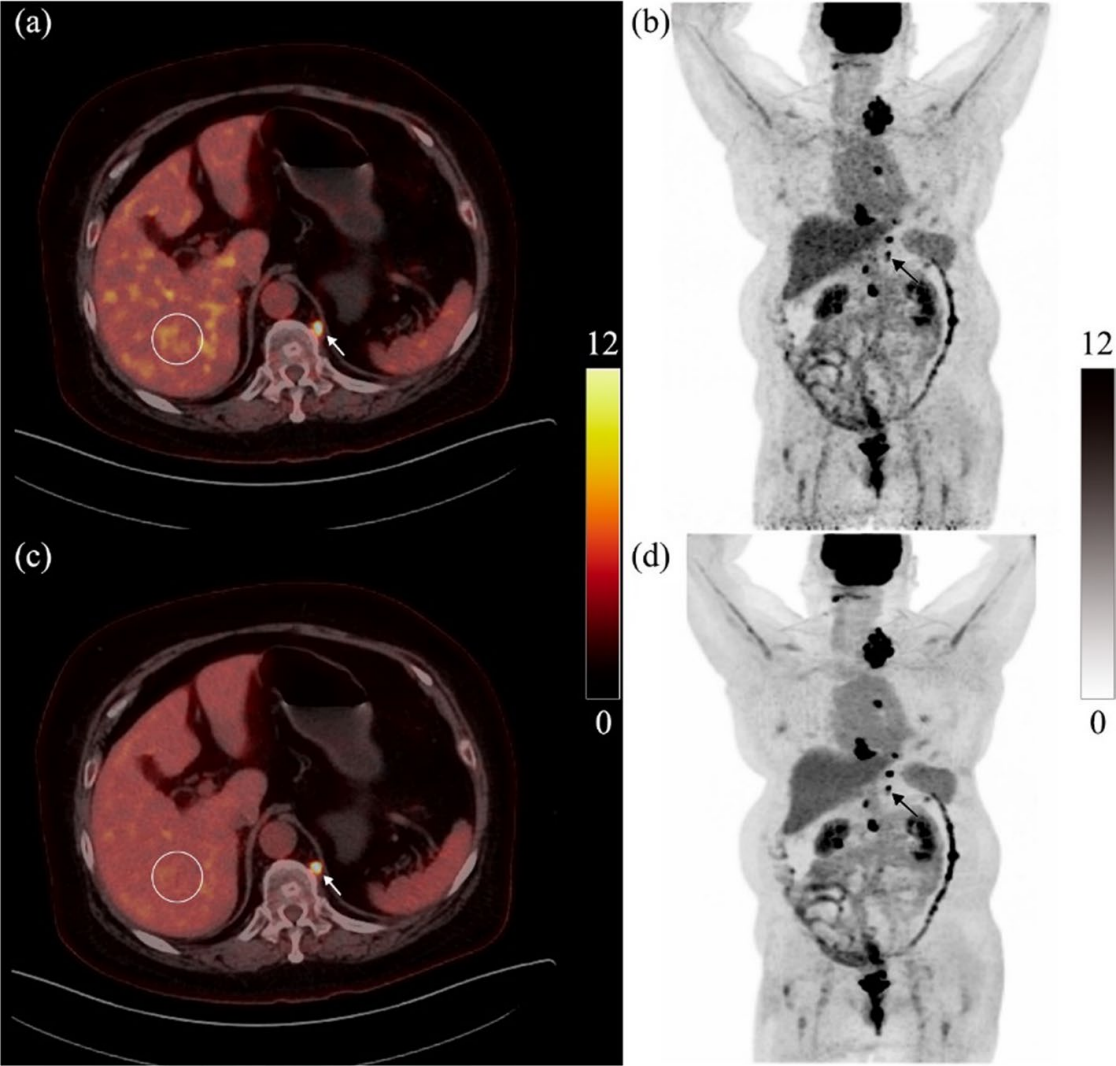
PET/CT image of an obese patient. a and c are PET/CT fusion images reconstructed by OSEM and DPL, respectively; b and d are MIPs reconstructed by OSEM and DPL, respectively. The circle on the liver was the VOI used to measure the data with a diameter of 3.0 cm. A metastatic para-aortic lymph node (seen the arrow) of 0.86 cm maximum diameter was detected by both methods. The SUV_max_ of this lymph node was 7.8 for OSEM and 9.0 for DPL
